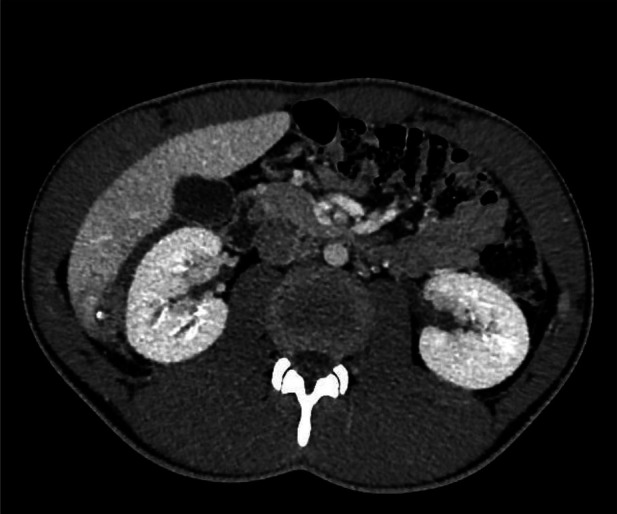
CT scan: Abscess in S6/S7 with an additional hyperdense calcified element.
